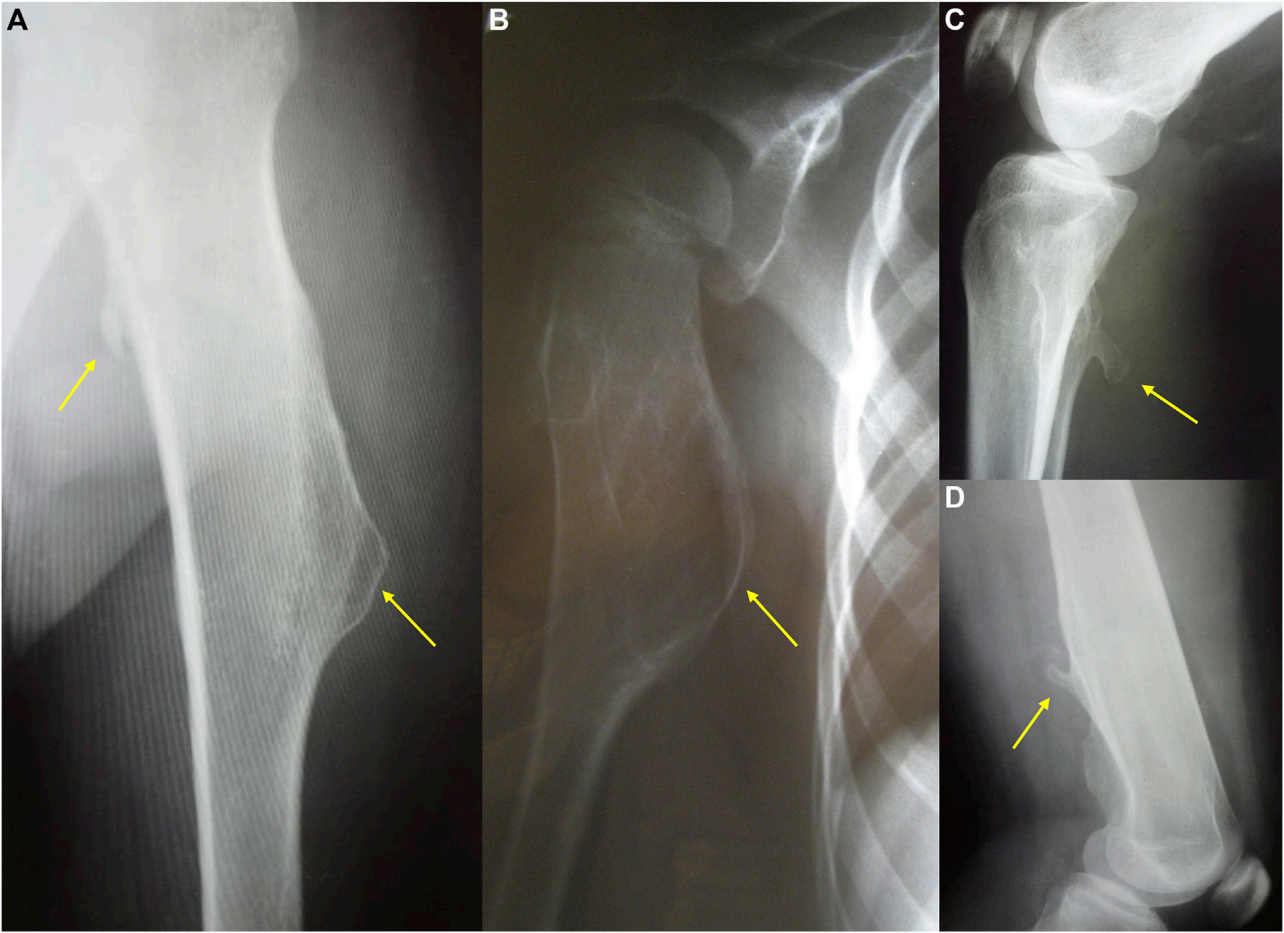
The radiography showing two different types of exostoses. Pictures (a,b) present sessile, mound shape osteochondromas, while (c,d) show pedunculated exostoses.
